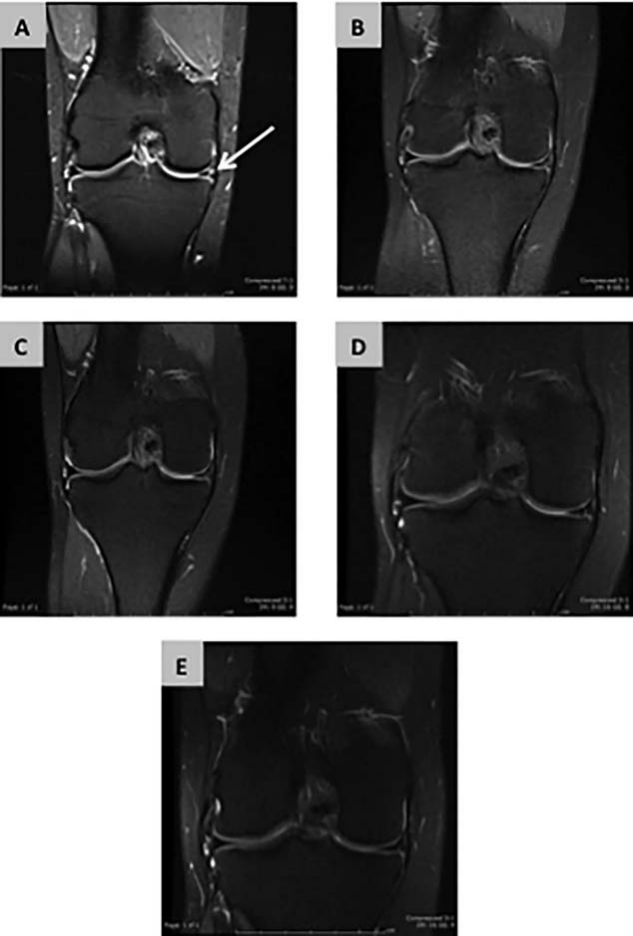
Magnetic Resonance Imaging (MRI) evidence of meniscal repair. Sequential coronal MRI images are show for one of the three patients (patient 3) who were successfully treated using human‐mesenchymal stem cells/collagen‐scaffold therapy. A Preoperative image is shown in (A) with an arrow to indicate the torn meniscus. Postoperative images were taken at 3 months (B), 6 months (C), 12 months (D), and 24 months (E). MRI data for the other four patients can be seen in Supporting Information Figures S8–S11.
